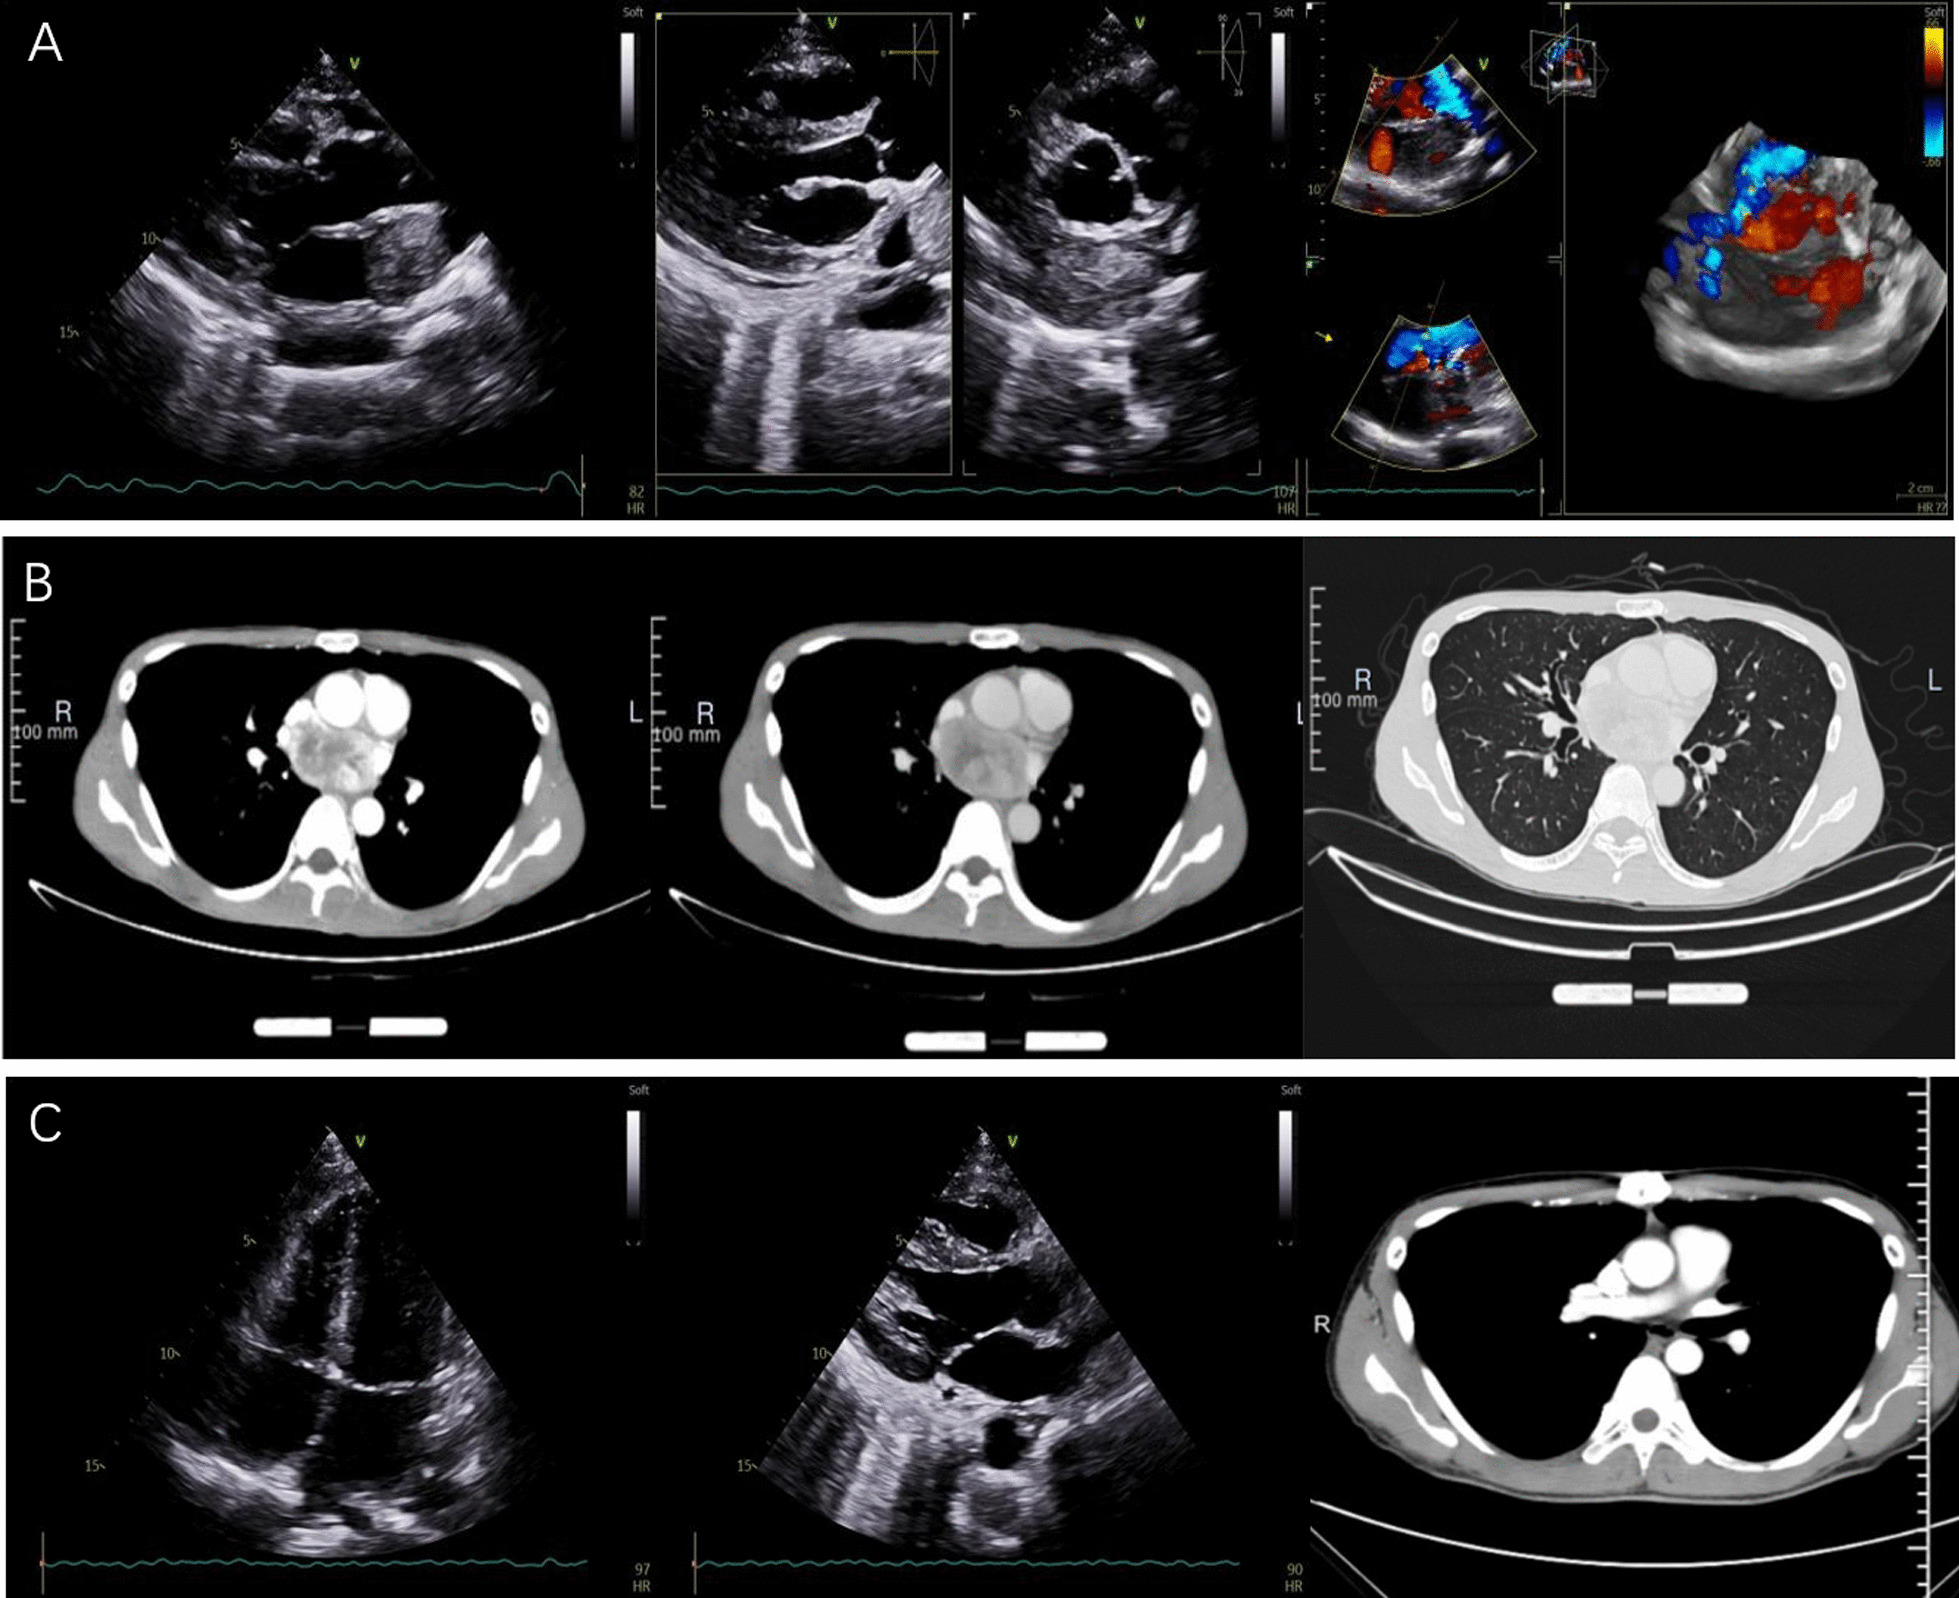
Preoperative imaging of the mass at transthoracic echocardiography (A) and CT (B). Postoperative transthoracic echocardiography and CT imaging (C)
